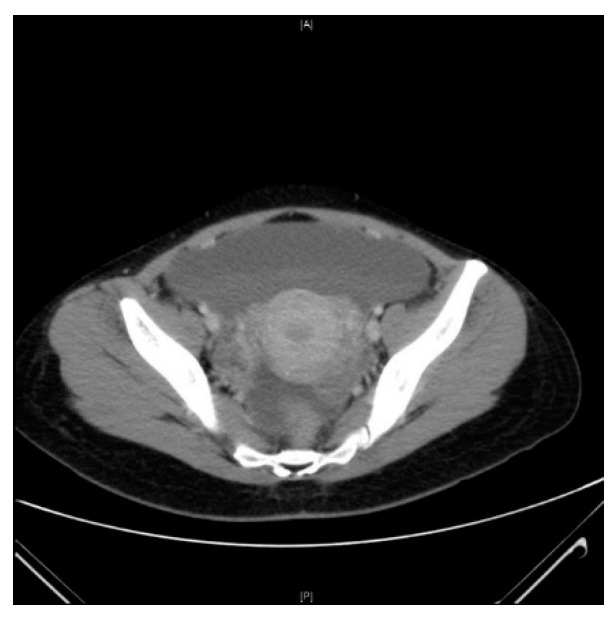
Computed tomography (CT) scan of pelvis demonstrating ascites with persistent left adnexal lesion.
